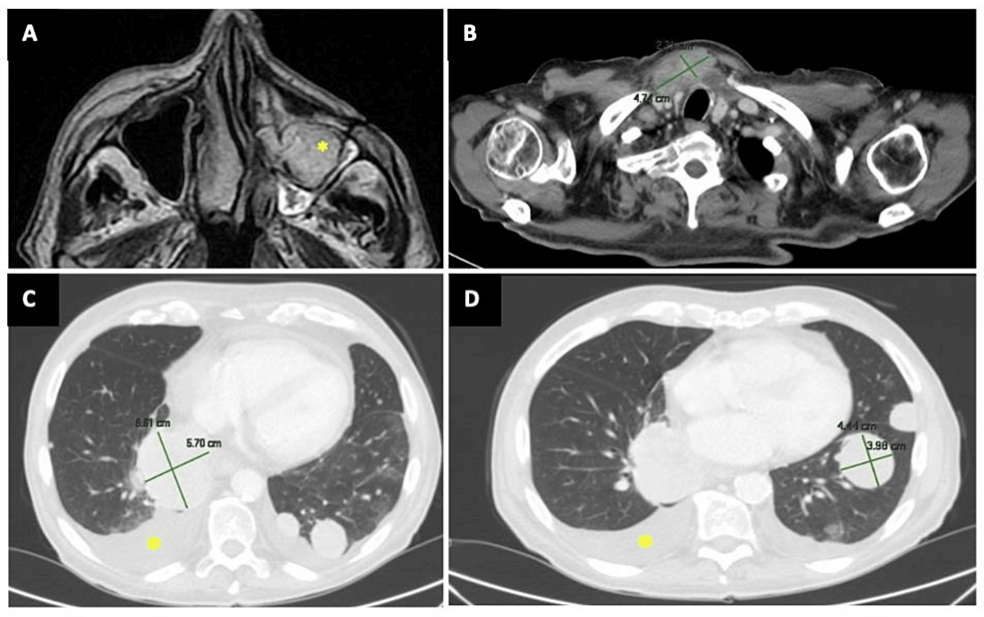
A. MRI shows an infiltrating mass in the maxillary sinus and nasal fossa (asterisk); B. Mediastinal lymphadenopathy measuring 4.7 x 2.2 cm in the jugular fossa; C and D. PET-CT image of lung metastases > 6 cm on the right side and > 4 cm on the left side, also pleural effusion (asterisk) PET: Positron Emission Tomography. CT: Computed Tomography. MRI: Magnetic Resonance Imaging
